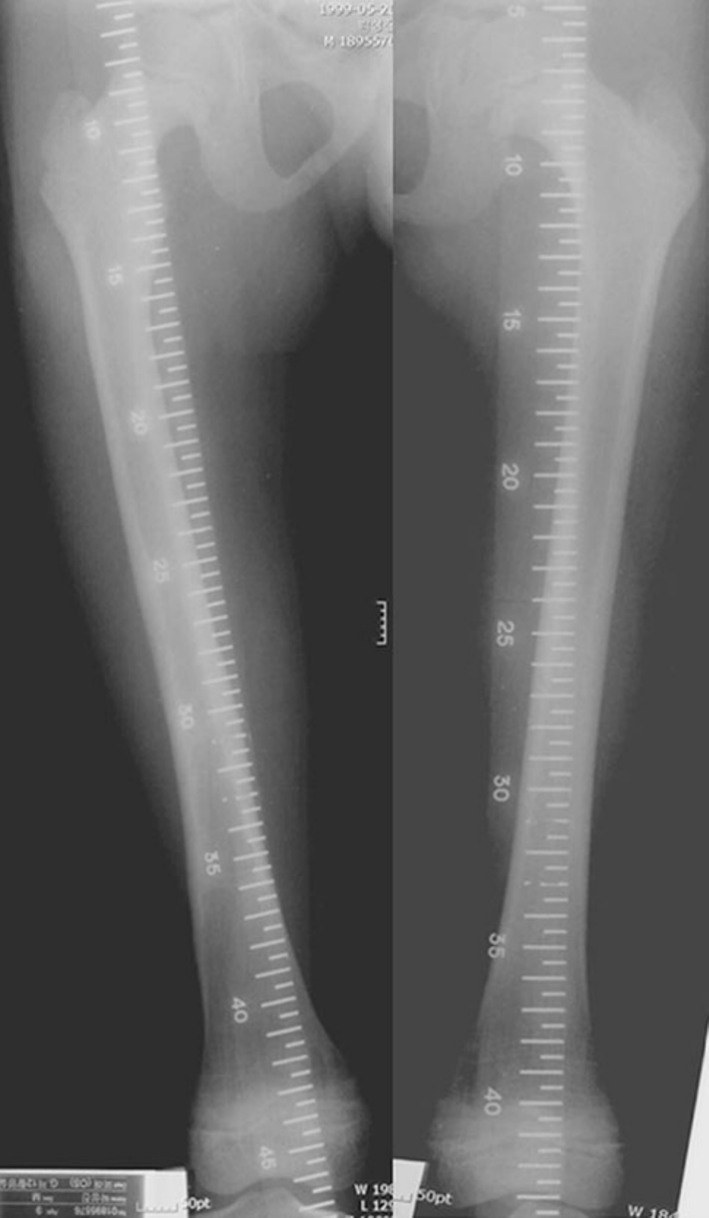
At two-year follow up, radiographs show overgrowth of the right femur of 1.5 cm
